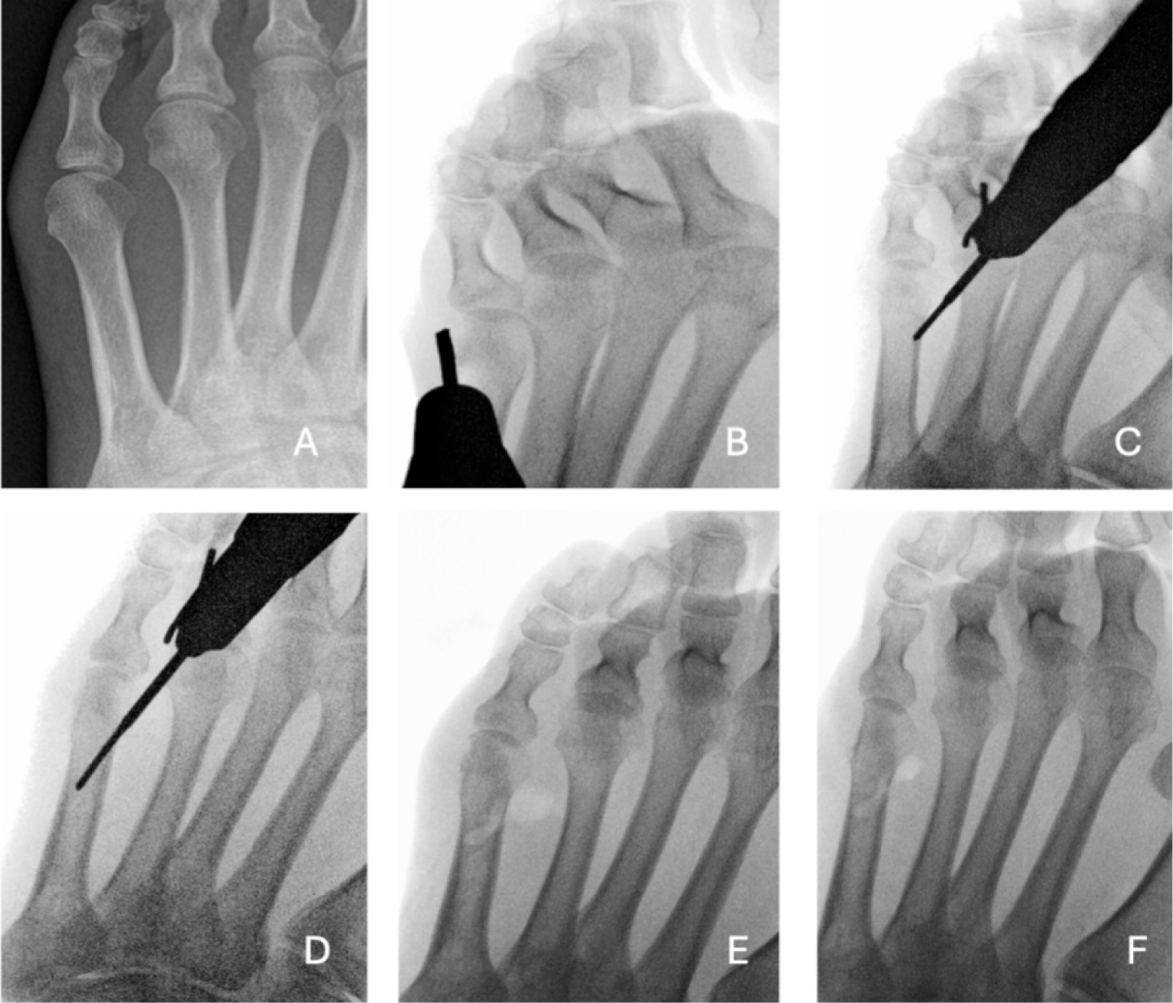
Pre- and intra-operative fluoroscopic sequence of bunionette correction using a Shannon burr. (A) Pre-operative anteroposterior radiograph showing lateral prominence of the fifth metatarsal head consistent with bunionette deformity. (B) Fluoroscopic image showing minimal lateral condylectomy performed using a Shannon burr. (C) Establishing the entry point for the osteotomy just proximal to the metatarsal head using the same burr. (D) The burr is advanced in a controlled manner to complete the osteotomy by cutting through both the dorsal and plantar cortices. (E) Completion of the osteotomy; the distal fragment is manually manipulated to achieve desired correction and closure. (F) Final fluoroscopic image showing the corrected alignment and final position of the fifth metatarsal following osteotomy and manual reduction.
